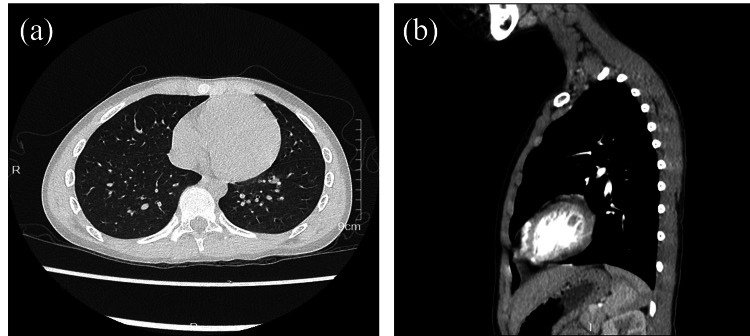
Contrast-enhanced chest CT findings showing no evidence of abscess formation. (a) Lung window: no abscess detected. (b) Mediastinal window: no evidence of mediastinal abscess formation.
